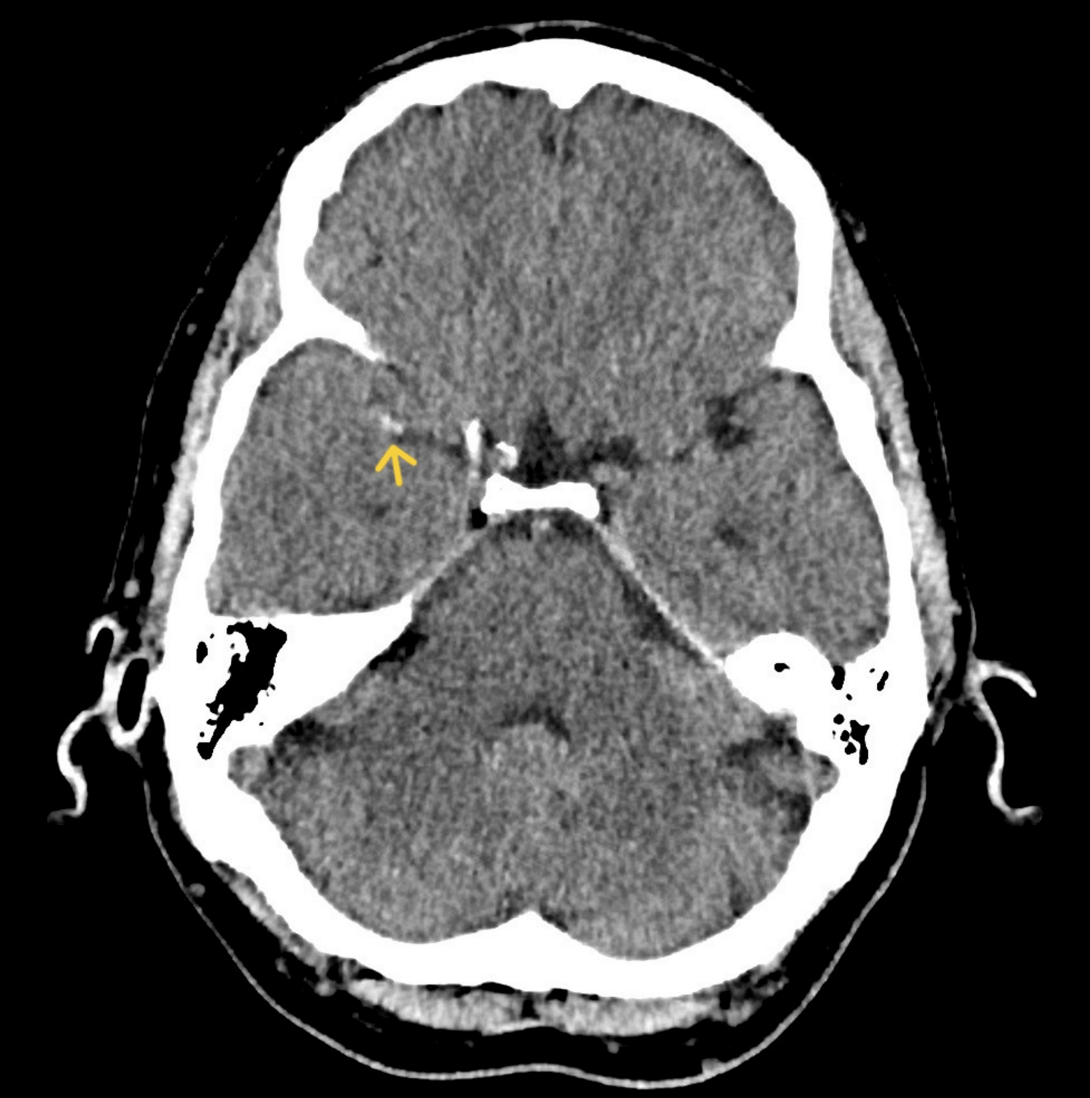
Non-enhanced brain CT scan showing M1 thrombus in the right middle cerebral artery. Yellow arrow pointing to the M1 thrombus.
